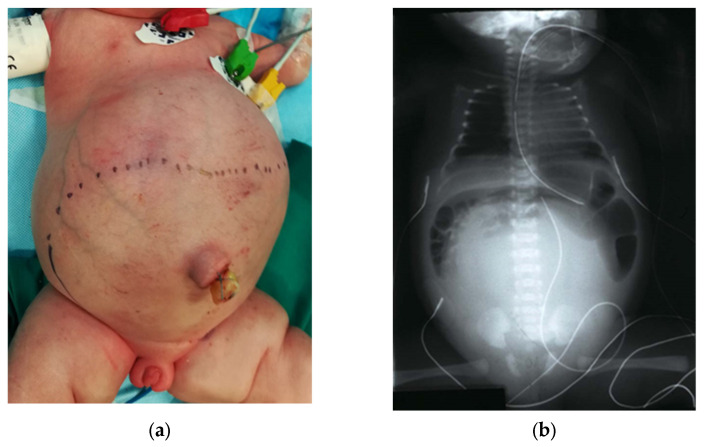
Hydrocolpos is found on: (a) a severely distended abdomen on physical examination at birth, and (b) a plain film radiograph showing a large opacifying structure with all bowel pushed superiorly, indicating a large pelvic mass.
